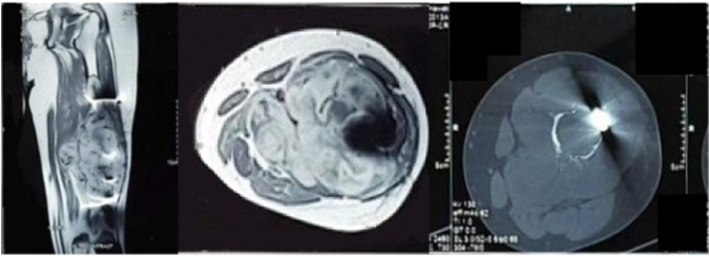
MR images showed tumor on femoral diaphysis with metal artifact.
